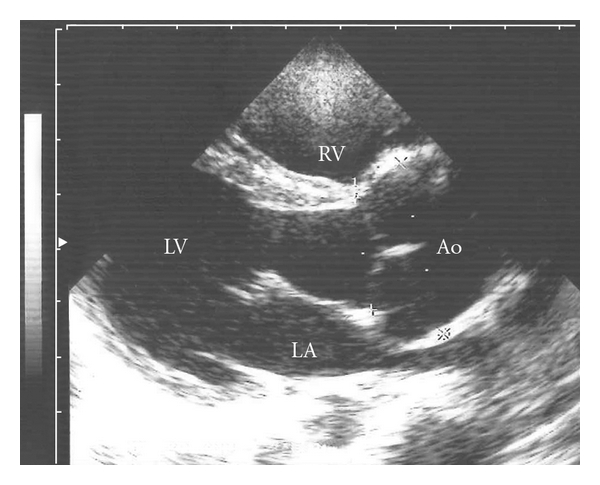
Parasternal long-axis view of left ventricle (LV) shows severe dilatation of the aortic root at the age of almost 4 years. Diameter of the aortic root is 32.2 mm. LA: left atrium; LV: left ventricle; RV: right ventricle; Ao: aorta.
